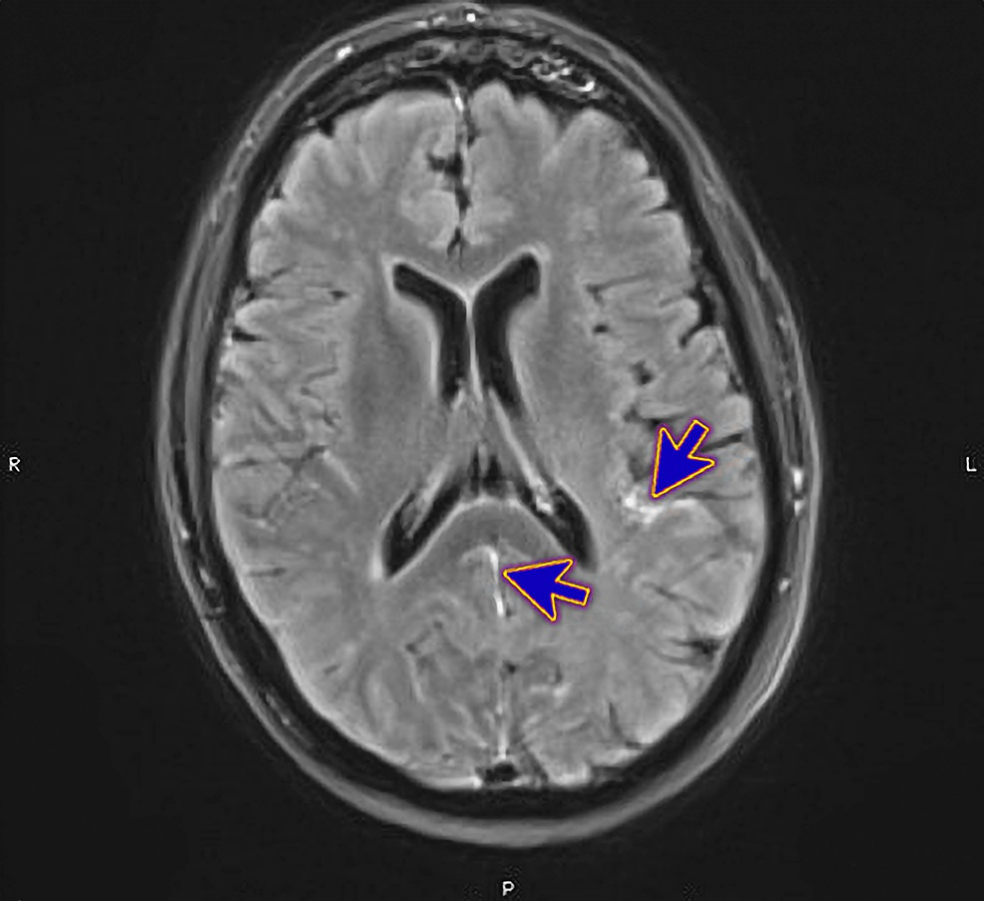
Magnetic Resonance Imaging (MRI) of the brain showing frontoparietal leptomeningeal enhancement along with interpedicular involvement with suspected cervical ependymal enhancement (blue arrows)
